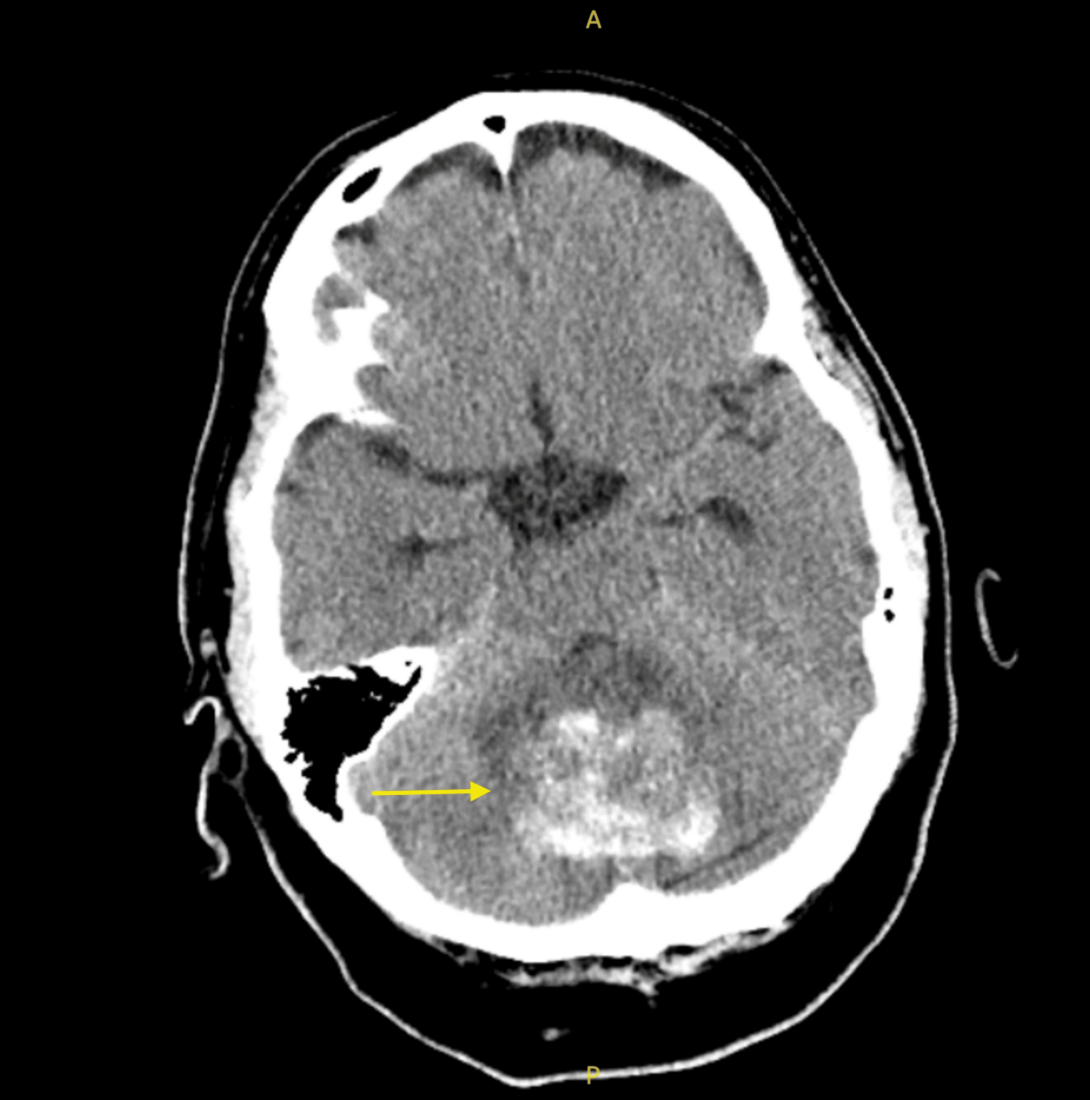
Axial non-contrast CT brain showing a 4.4 cm hemorrhagic cerebellar mass (yellow arrow) with mass effect on the fourth ventricle and early hydrocephalus The letters "A" and "P" indicate the anterior and posterior aspects of the image, respectively. Image Credit: Daniel Jones
